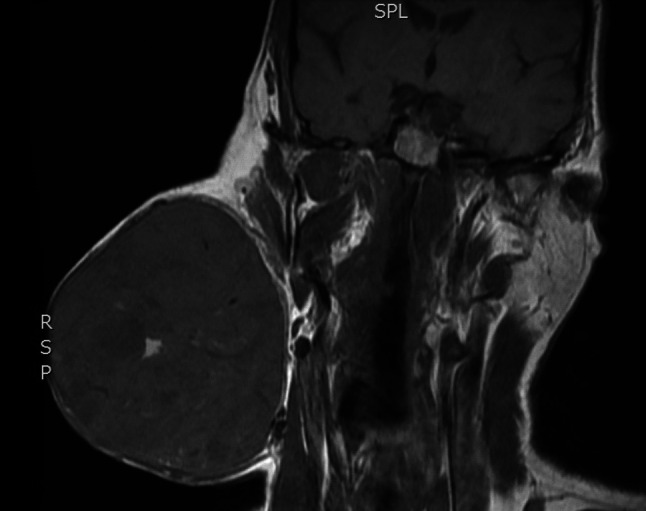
Coronal MRI neck - showing tumour pre-operation
